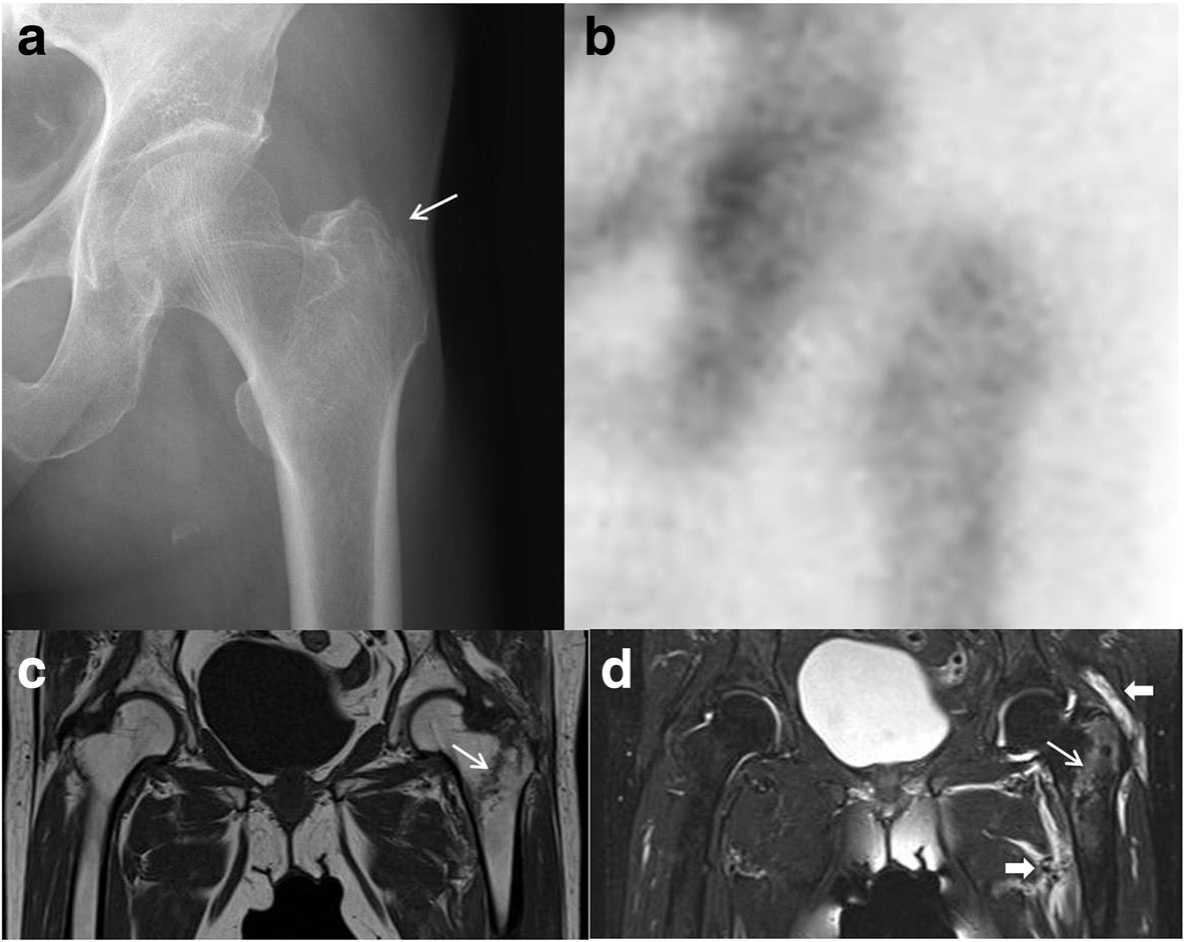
Case of an 84-year-old woman who presented at the emergency department with left hip pain after slipping in the bathroom. a A plain radiograph of the left hip only shows an isolated greater trochanter fracture (*arrow*). b A bone scan obtained 2 days after the trauma was unremarkable. c The T1-weighted coronal image shows an intertrochanteric fracture (*arrow*) that extends to the medial cortex. d The T2-weighted coronal image shows a fracture at the same level (*arrow*) and abnormal signal in the muscles around the hip (*bold arrows*)
